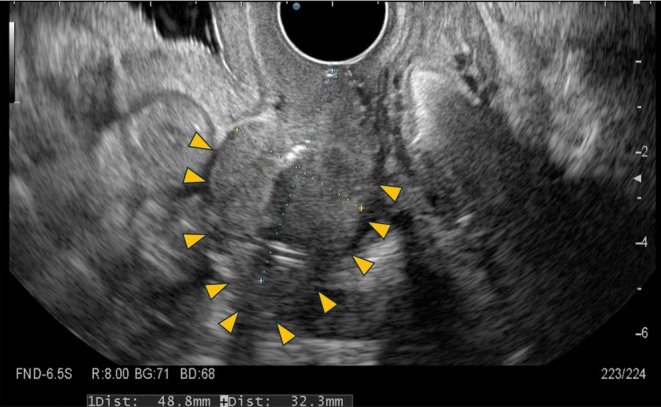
Transvaginal ultrasonography 2 months after ethanol sclerotherapy No echo‐free space was observed in the uterine cavity, and similar findings persisted thereafter. A linear hyperechoic band along the endometrial surface suggests intrauterine adhesion formation that likely prevented recurrence. Yellow arrowheads: Outline of the uterine body.
